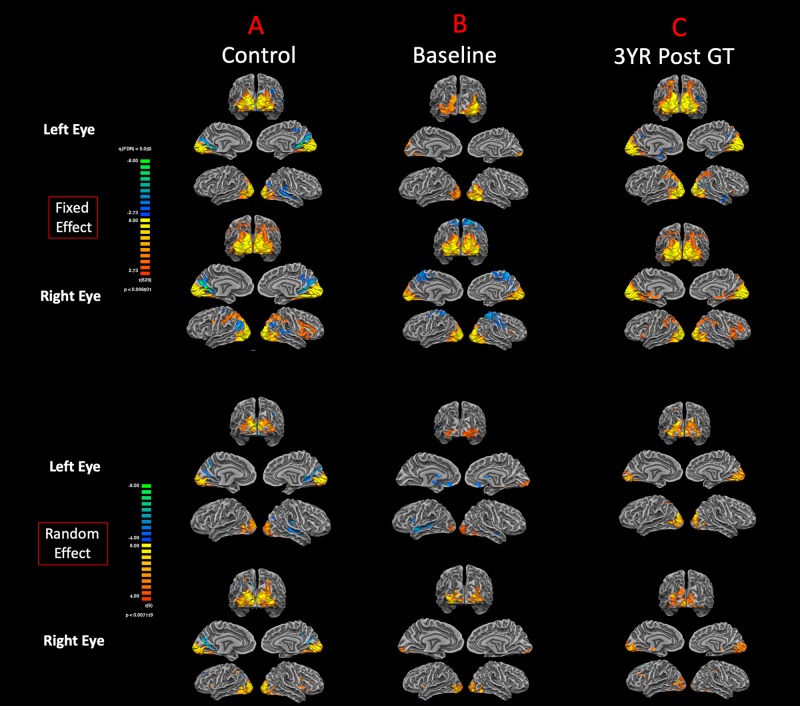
Functional magnetic resonance imaging response to visual stimulation. The visual stimulation tasks were presented either to the left or right eye of (A) normal-sighted controls, (B) RPE65 patients before GT, and (C) RPE65 patients 3 years after GT. All task-based cortical activations were processed using FX analyses at FDR corrected *p* < 0.007 (*q* < 0.05), with an extent threshold of >100 mm^2^ and RFX analyses at an uncorrected *p* < 0.007 with an extent threshold > 100 mm^2^. Sighted controls showed significant bilateral cortical activations in the visual cortex. At baseline, RPE65 patients expressed asymmetric and attenuated hemispheric activations in the left eye because the left eye was untreated in seven of eight patients. However, visual stimulation of the right eye in RPE65 patients displayed nearly symmetrical bilateral activation of the visual cortex. Three years post–FO clinical trial when RPE65 patients’ contralateral eye was treated, symmetrical bilateral visual cortex activations were observed for both left and right eyes.
